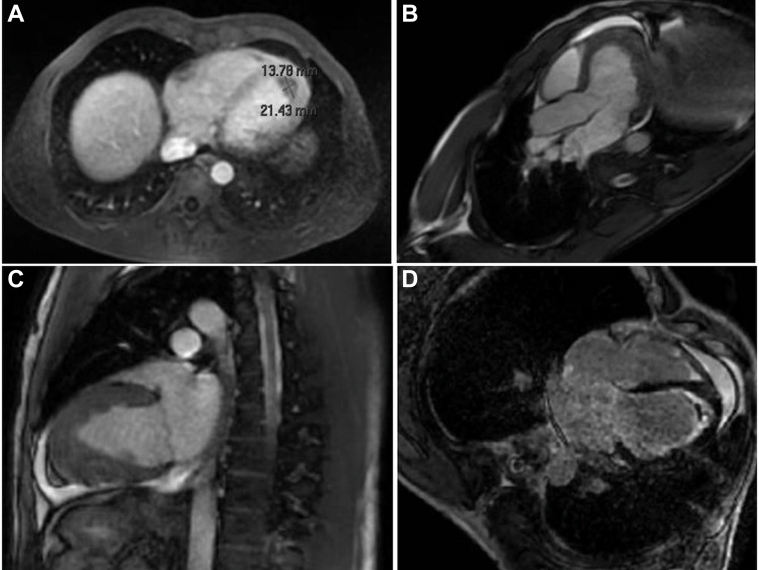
Baseline Cardiac Magnetic Resonance Images (A) Axial late gadolinium enhancement (LGE) image showing an apical thrombus in the left ventricle (LV) measuring 14 × 21 mm. (B and C) Cine SSFP sequences in the apical 3-chamber (B) and apical 2-chamber (C) views demonstrating an LV with apical thickening/obliteration due to intracavitary fibrotic endomyocardial tissue. (D) LGE study in the apical 4-chamber view showing circumferential subendocardial enhancement involving the apical and midventricular segments of the LV, with right ventricular involvement (apical thickening and a laminar subendocardial thrombus without enhancement) displaying the same enhancement pattern. SSFP = steady-state free precession.
